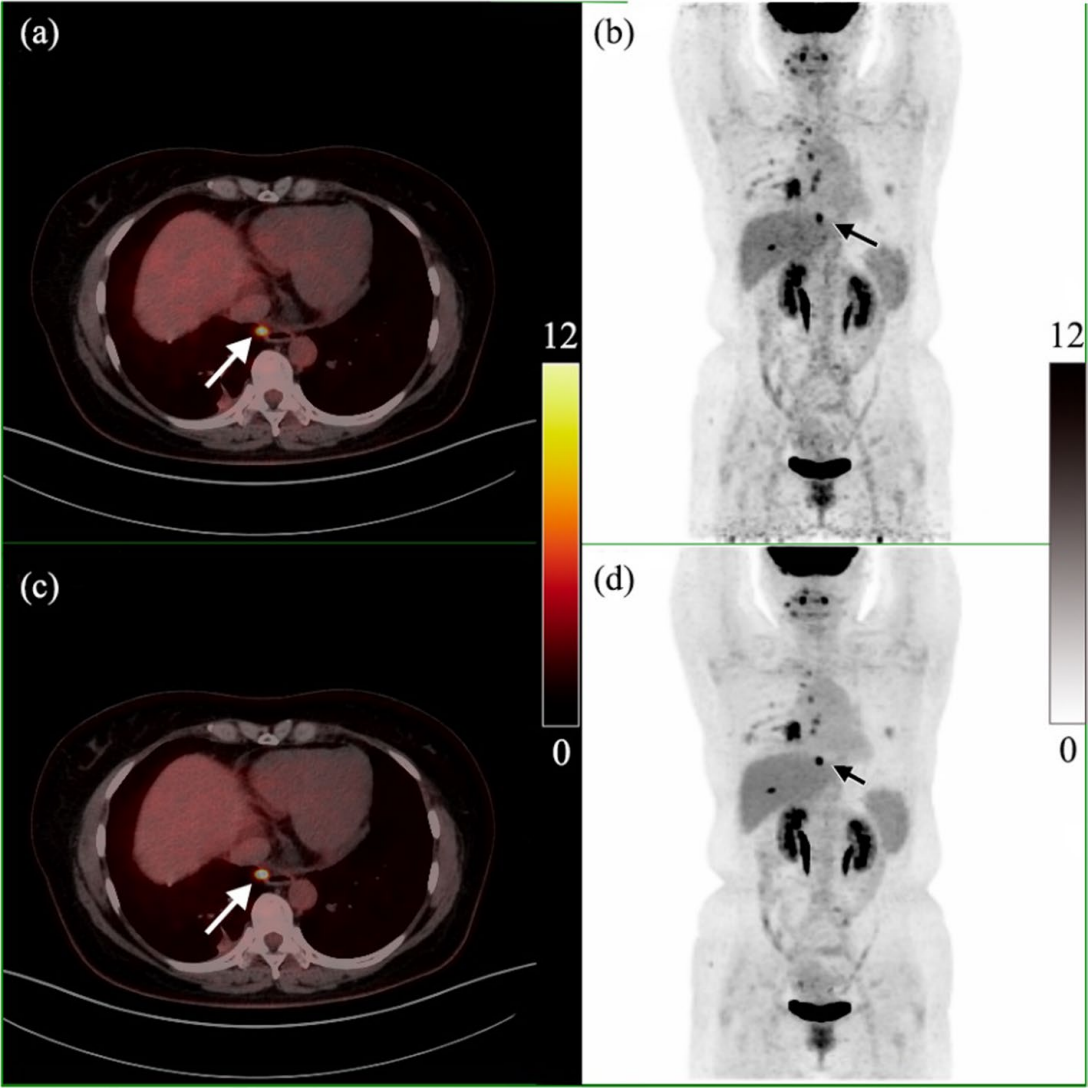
PET/CT image of a patient who was diagnosed with the adenocarcinoma of lung. a and c are PET/CT fusion images reconstructed by OSEM and DPL, respectively; b and d are MIPs reconstructed by OSEM and DPL, respectively. The SUV_max_ of the mediastinal lymph node (seen the arrow) with a maximal diameter of 0.67 cm was 6.5 for OSEM, and 8.8 for DPL
